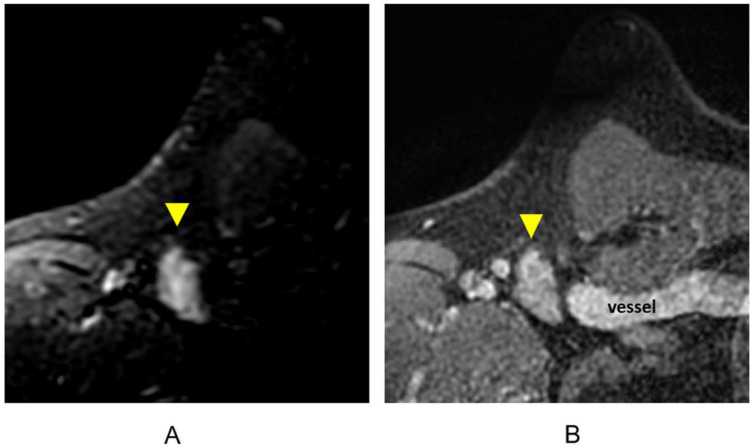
Lymph node (arrowheads). (A) Axial STIR sequence; (B) axial post-contrast T1-weighted fat-suppressed sequence.
